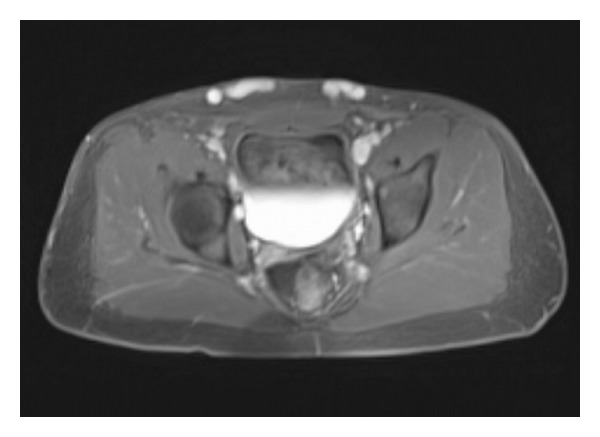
Axial MRI of pelvis with contrast showing large suprapubic varicosities (white arrows) and a small right external iliac (short arrows). The left external iliac vein is larger (long arrows).
